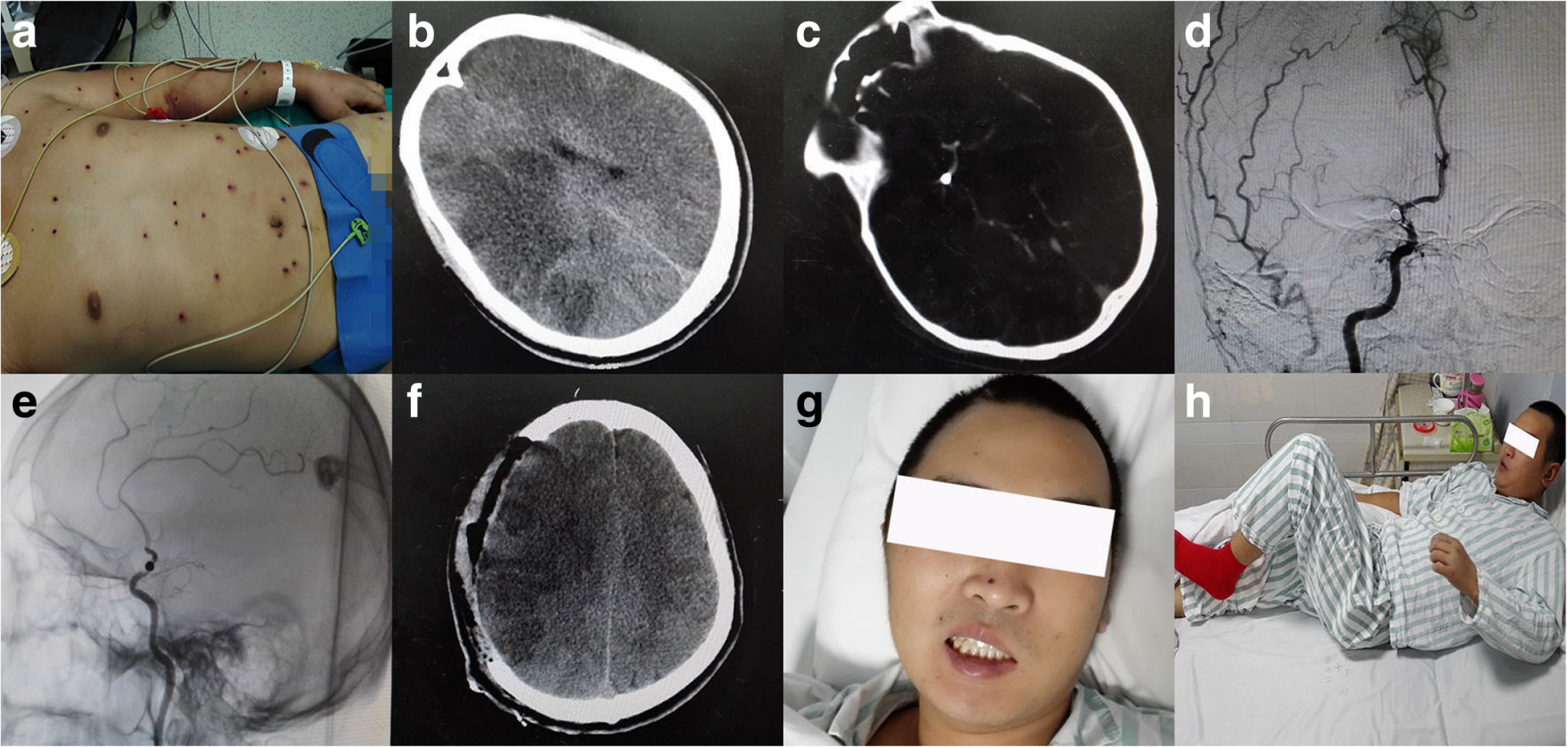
Picture and CT image of the patient. The patient with gunshot wounds showed recovery of the intracranial blood supply and physical ability gradually due to our contribution of surgery.a Multiple gunshot wounds in the skin of this patient; b CT scan revealing large parts of low-density shadows in the right frontal lobe, right parietal lobe and right temporal lobe before the surgery; c CT scan revealing the pellet lodged in the brain; d, e DSA showed the branches after the initial part of the right middle cerebral artery (MCA) were obliterated; f CT scan showed decreased low-density areas with the midline moving back 24 h after the surgery; g Posterior-anterior picture of the patient 2 weeks after the surgery showed a decreased difference between two sides of the face; h The patient left the hospital with myodynamia degree III of his left upper limb, degree V of the proximal part of his left lower limb and degree V of the distal part of his left lower limb
